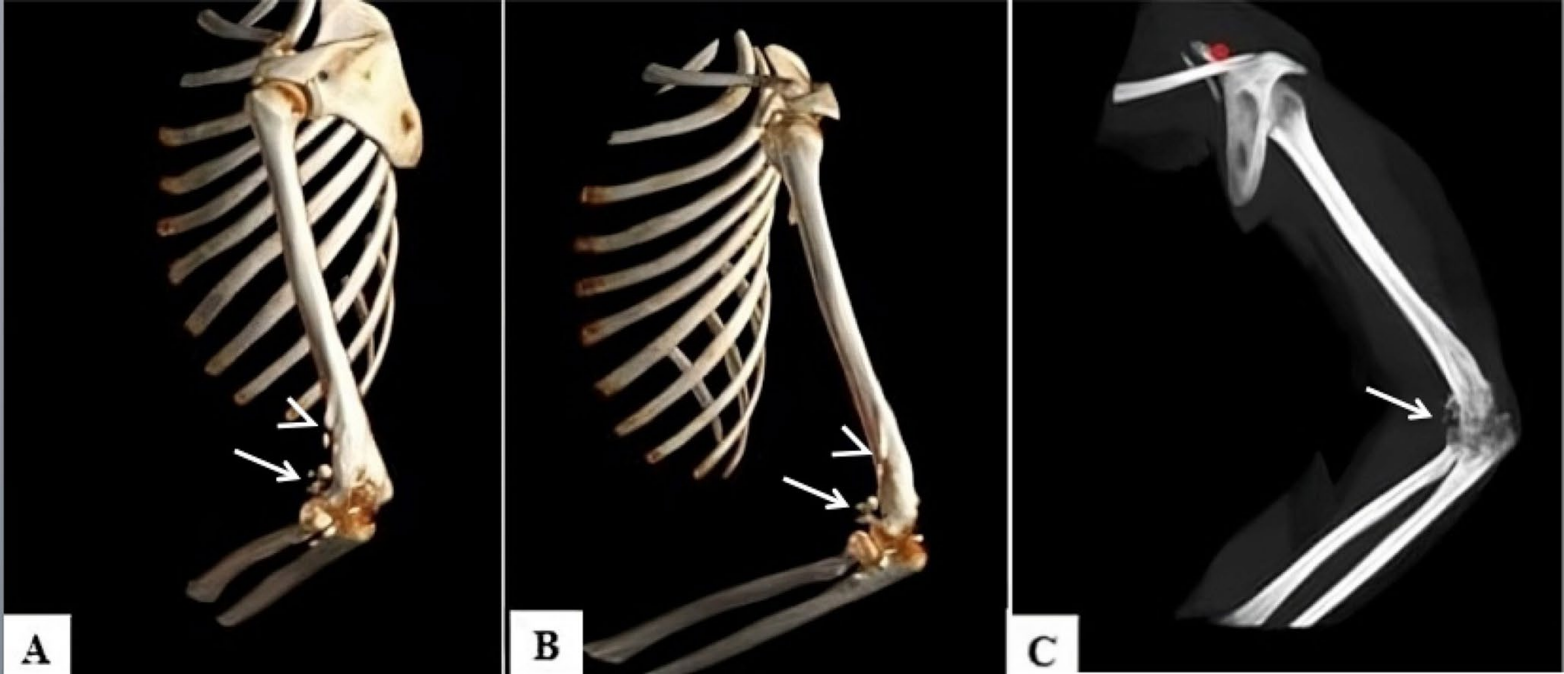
Victim of torture due to blunt force injuries in the upper limb and signs of deformation. CT with volume rendering reconstructions (lateral projection A, anteroposterior projection B) and maximun intensity projection (anteroposterior projection C) shows: structural deformity as results of complex fracture with alteration of the mid-distal third of the humerus, the humeral epicondyles, the ulnar olecranon, and the proximal epiphysis of the radius (especially the coronoid process); bone fragments coexist in the context of the surrounding soft tissues (white arrow). Also note marginal osteophytosis likely due to a post-traumatic arthritic process on a degenerative basis (white arrowhead)
